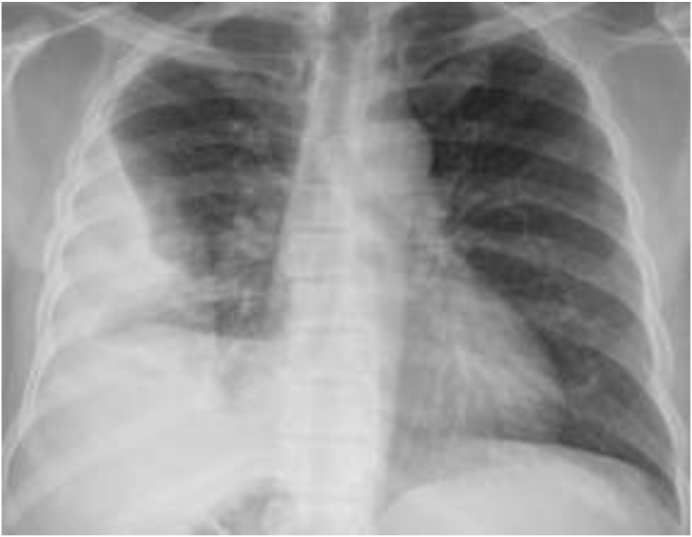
Chest X-ray showing right-sided moderate effusion with thickening.
